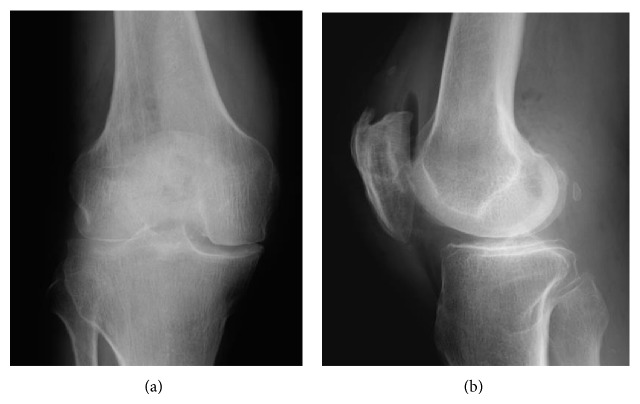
Plain radiographs after surgery to remove all wires.
